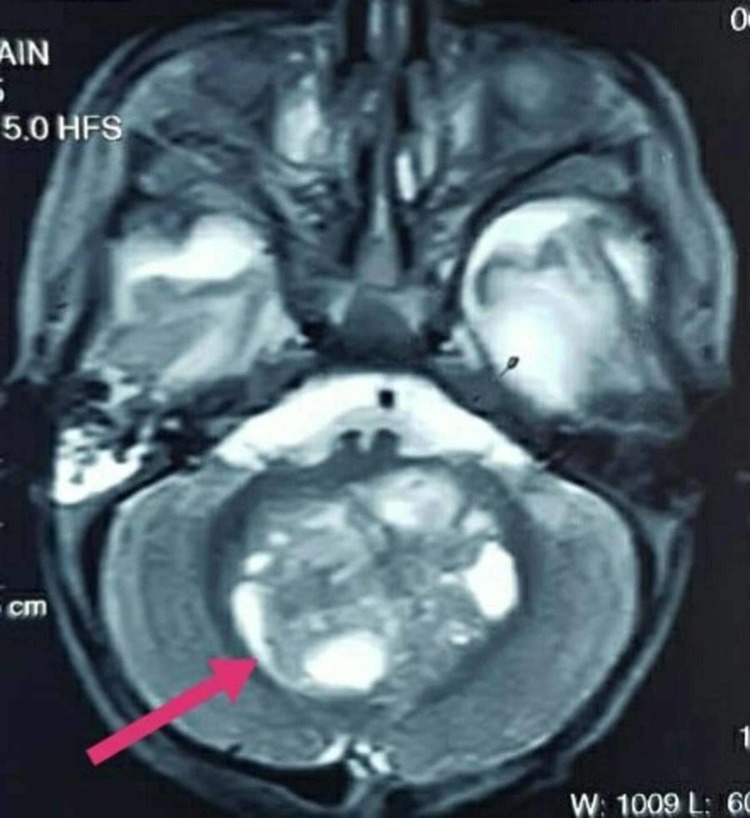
Magnetic resonance imaging of the brain showing a round heterogeneous mass in the posterior fossa along midline
